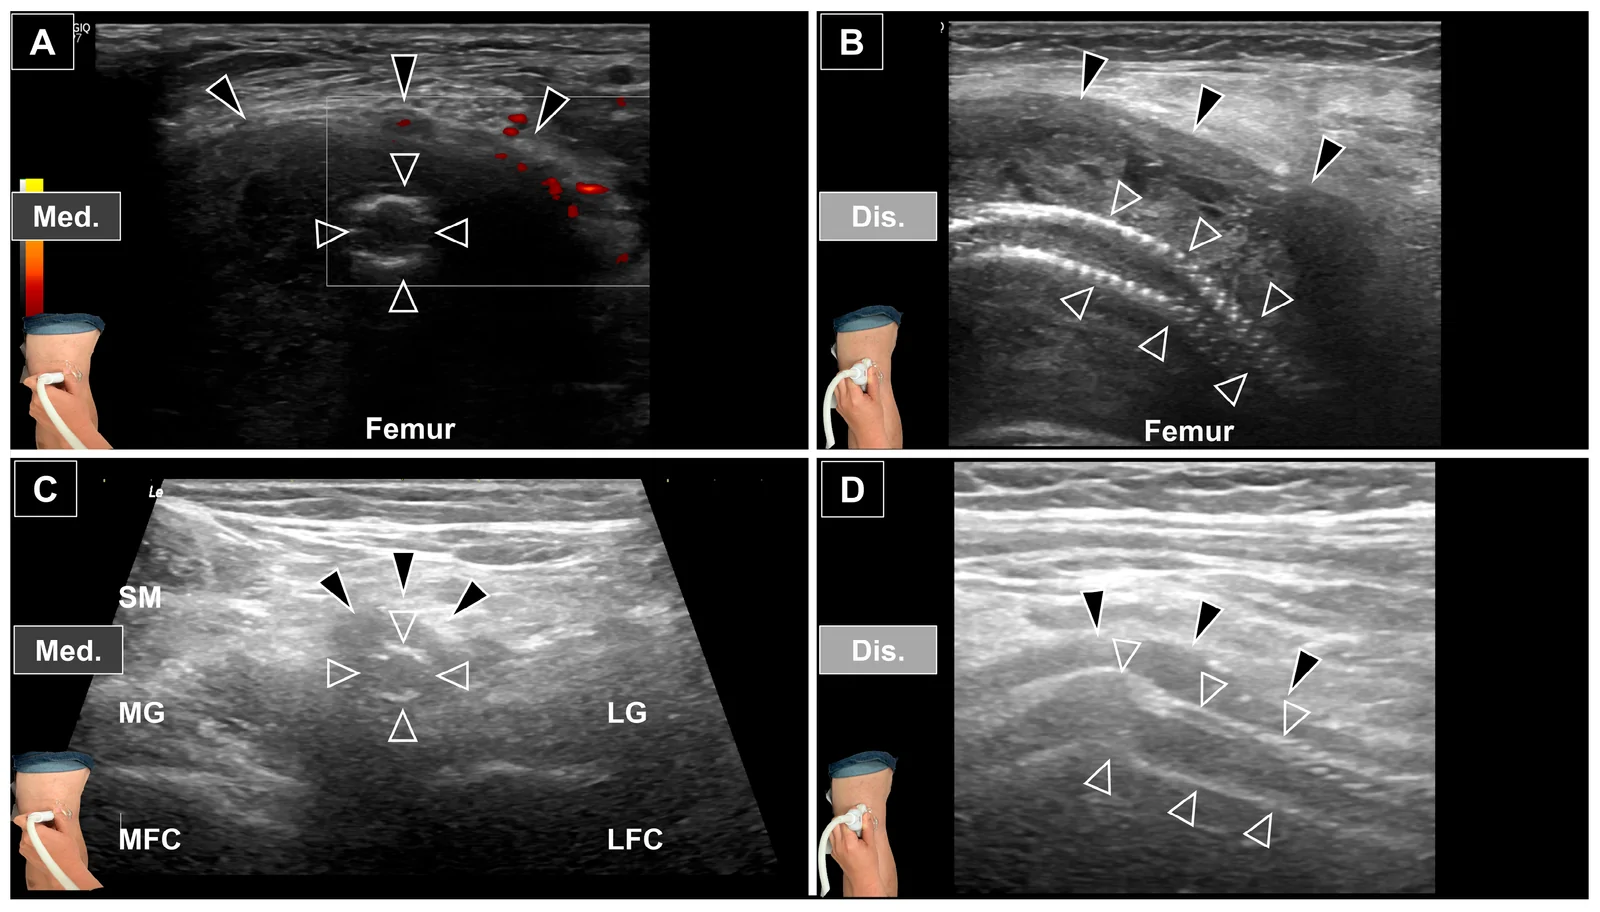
Serial ultrasonographic follow-up at three and 18 months following endovascular exclusion of a thrombosed popliteal artery aneurysm. Panels (A,B) show the short-axis and long-axis views at three months, whereas panels (C,D) show the corresponding views at 18 months. The white open arrowheads indicate the stent graft, which remains structurally stable and patent without sonographic evidence of re-occlusion [4,8]. The black arrowheads indicate the remaining aneurysmal sac with heterogeneous hypoechoic thrombotic content. Compared with the three-month examination, the 18-month surveillance shows progressive remodeling of the remaining aneurysm, with reduced thrombus burden and decreased mass effect on adjacent neurovascular and muscular structures [2,4]. Clinically, the preoperative right foot paresthesia improved progressively over the first 3 months after endovascular repair, in parallel with the sonographic findings. Secondary surgical decompression was not required. Decompression remains appropriate when neurological symptoms are attributable predominantly to persistent mass effect, as has been described for other peripheral arterial aneurysms [12,13]. In this patient, however, symptom relief followed both restoration of arterial inflow and progressive reduction in sac volume, and the relative contribution of chronic ischemia and local compression therefore cannot be separated. During long-term aspirin monotherapy, serial ultrasound at 18 months confirmed sustained stent-graft patency and progressive aneurysmal sac remodeling. Secondary interventions have been reported in approximately 33% to 63% of patients within two years of popliteal artery aneurysm repair [4], and long-term imaging surveillance is therefore warranted. Ultrasonography is well suited to this task, since it requires neither contrast nor ionizing radiation and can be repeated at the bedside to assess stent-graft patency, sac remodeling, and recurrent disease. By documenting both durable stent-graft stability and progressive aneurysm sac remodeling, this 18-month ultrasound follow-up complements the initial multimodal assessment and underscores the value of longitudinal imaging follow-up in this atypical presentation [5]. Abbreviations: Dis., distal; Med., medial.
